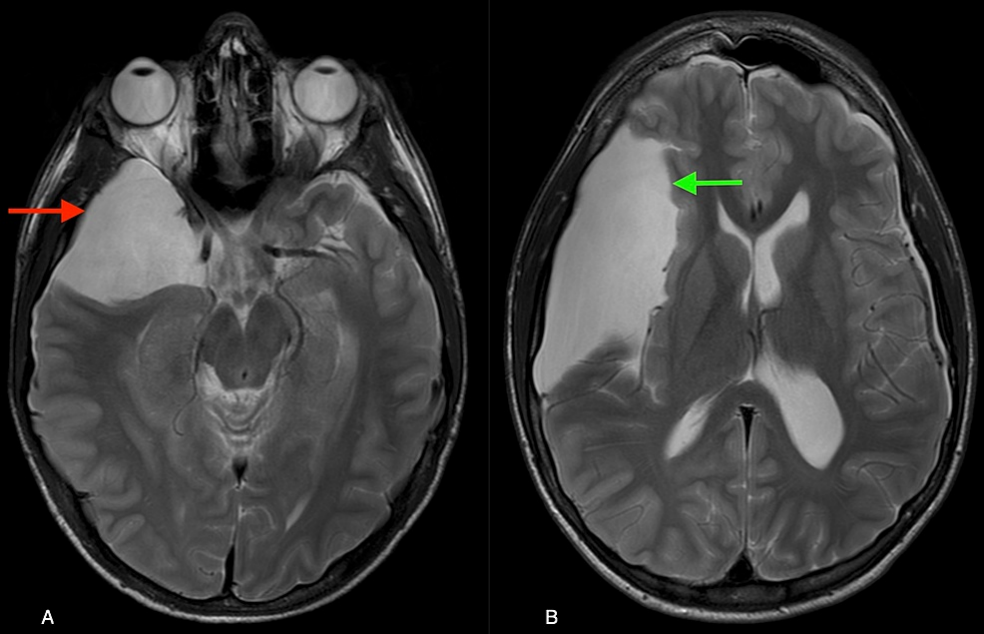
Axial T2 MRI brain at the level of the midbrain (A) and basal ganglia (B) demonstrating a large right middle fossa arachnoid cyst (red arrow) extending into the right sylvian fissure (green arrow).
